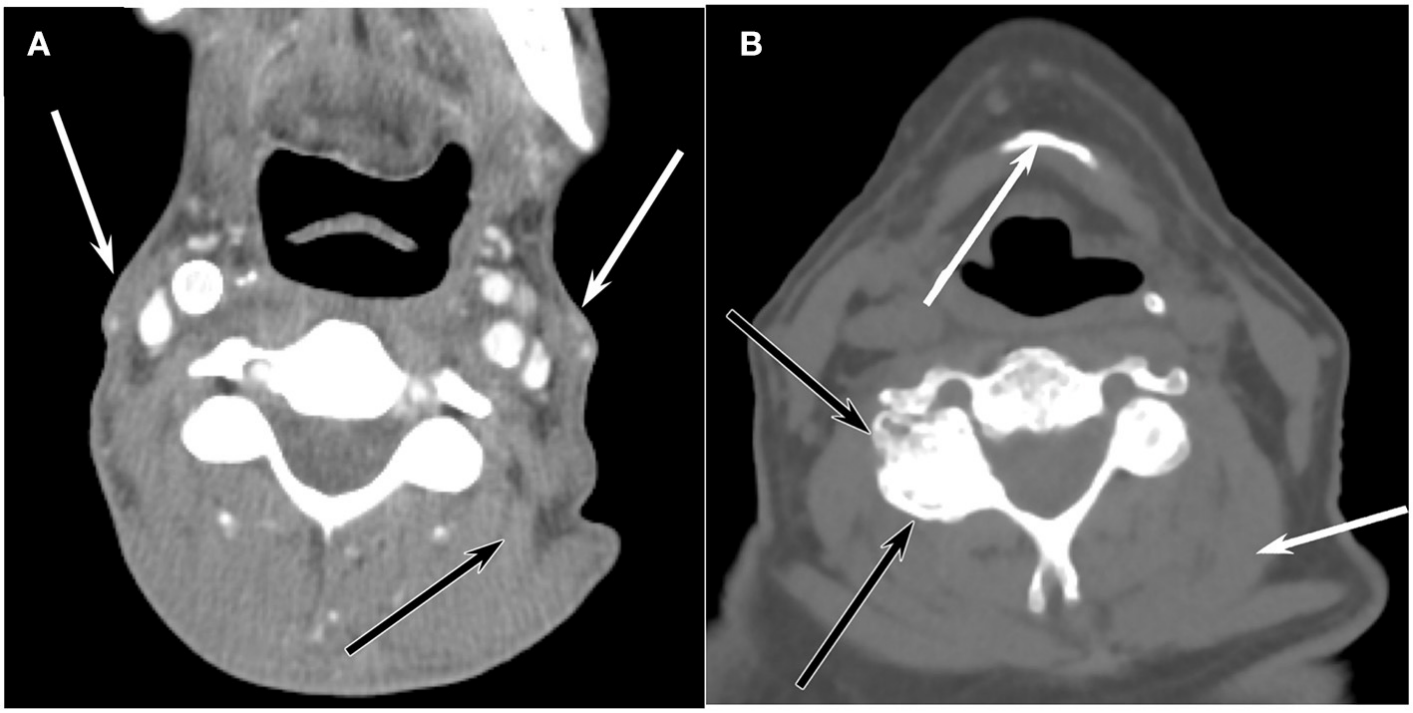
Axial CT representations of (A) Enhanced axial CT of a 56-year-old man at the level of C3 shows marked atrophy of the sternocleidomastoid muscles (white arrows) and paraspinal muscles such as the levator scapulae muscle (black arrow), but there is no appreciable degenerative disease in the facet joints or discs. (B) Unenhanced axial CT image at the level of C3 reveals extensive facet arthrophy (black arrows) and disc disease, but the muscle volume (white arrow) is normal for a 71-year-old man.
